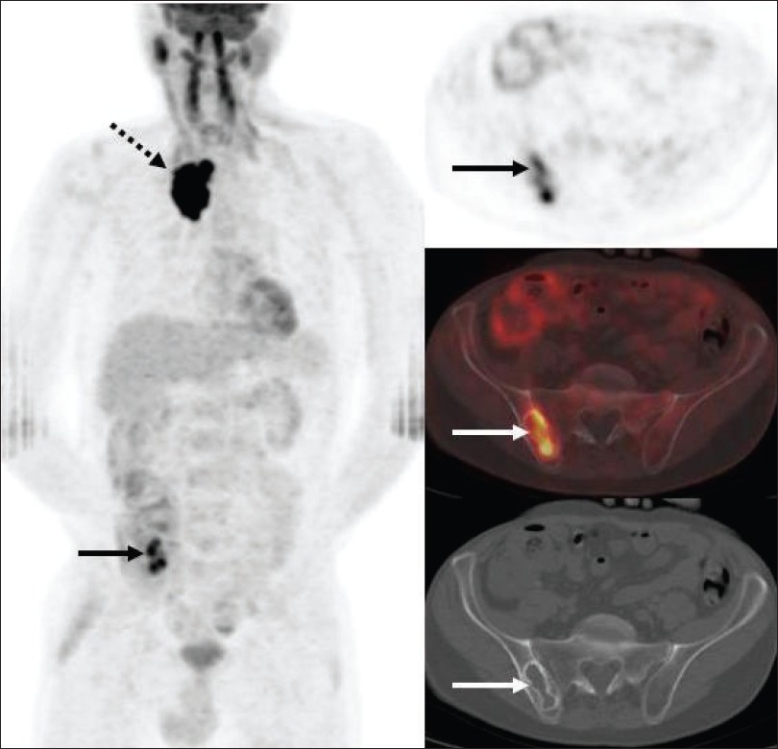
Axial PET (top right), fused PET/CT (middle right) and CT (bottom right) images at the level of the pelvic inlet, with an MIP image (on left), from a PET study of a 71-year-old man with a right upper lobe cancer. In addition to showing intense uptake in the right upper lobe lung cancer (dotted arrow), the study also shows an unsuspected right iliac bone metastasis, upgrading the patient to stage IV disease
